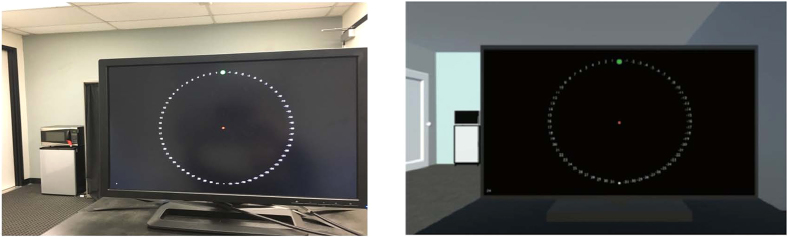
Experimental environments. Top: Visuomotor adaption task in conventional training (CT). Bottom: Visuomotor adaptation task in head-mounted virtual reality (HMD-VR). The environment in virtual reality was designed to emulate the environment of the conventional training condition in the size and lighting of the room, the furniture placed around the room such as a refrigerator and a desk, the computer monitor, and more.
